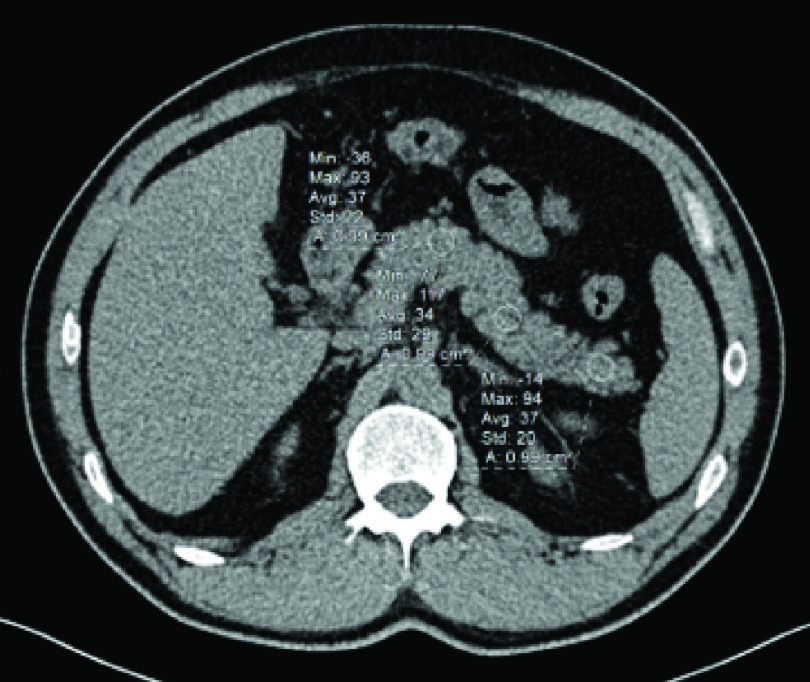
Unenhanced axial computed tomography image of a 43-year-old male patient with COVID-19. Five different ROIs (using a circular ROI of 1 cm^2^) were drawn over five anatomical parts of the pancreas to assess the attenuation value of the organ by taking the average of the 5 HU values collected from the ROIs. Shown here are the measurements from the neck, body and tail of the pancreas (37, 34 and 37 HU, respectively). The average attenuation value of the pancreas was 35 HU in this patient. HU: Hounsfield unit; ROI: Region of interest.
